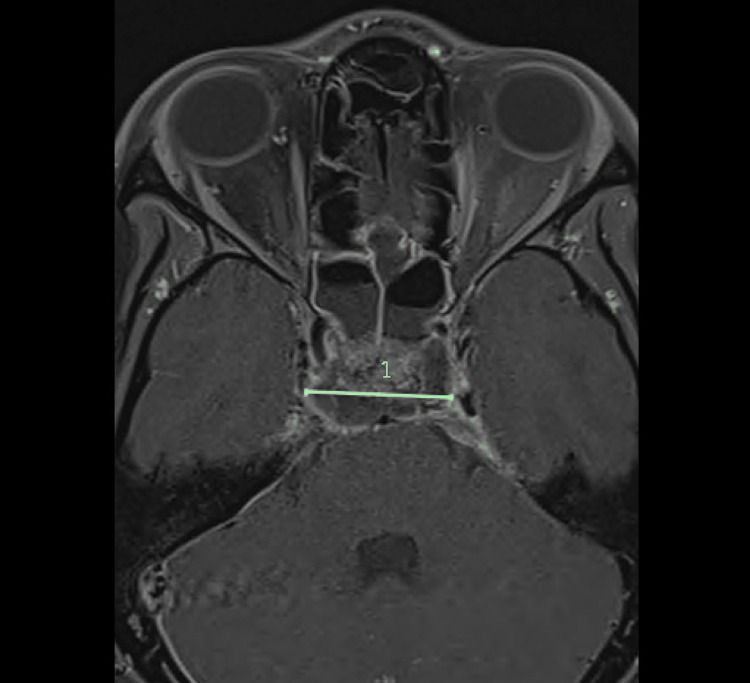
MRI of the brain/orbit with contrast, axial cut, 3.3 cm transverse abscess (scale) at the dorsal aspect of the sella and clivus with involvement of the cavernous sinus bilaterally MRI, magnetic resonance imaging.
